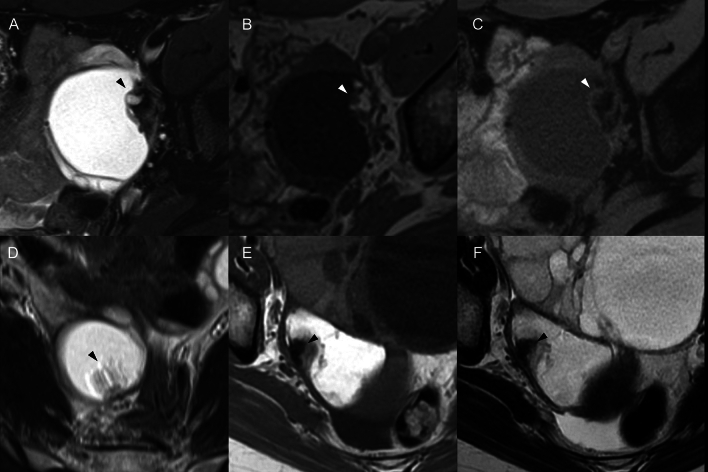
A mural nodule in benign mature cystic teratoma. a–c A 27-year-old woman. Axial fat-suppressed T2-weighted image (T2WI) a shows a hyperintense mass with a heterogeneous mural nodule along the left side wall of the mass (arrowhead), axial T1-weighted image (T1WI) b exhibits the mural nodule with internal hyperintensity, and on axial fat-suppressed T1WI c, the hyperintensity within the mural nodule on T1WI changes to a hypointensity, indicating a fat component. d A 28-year-old woman. An axial T2WI displays a palm tree-like structure projecting on the hyperintense mass (arrowhead). e, f A 36-year-old woman. Axial T1WI e and T2WI f reveal a signal void indicative of a tooth-like structure
